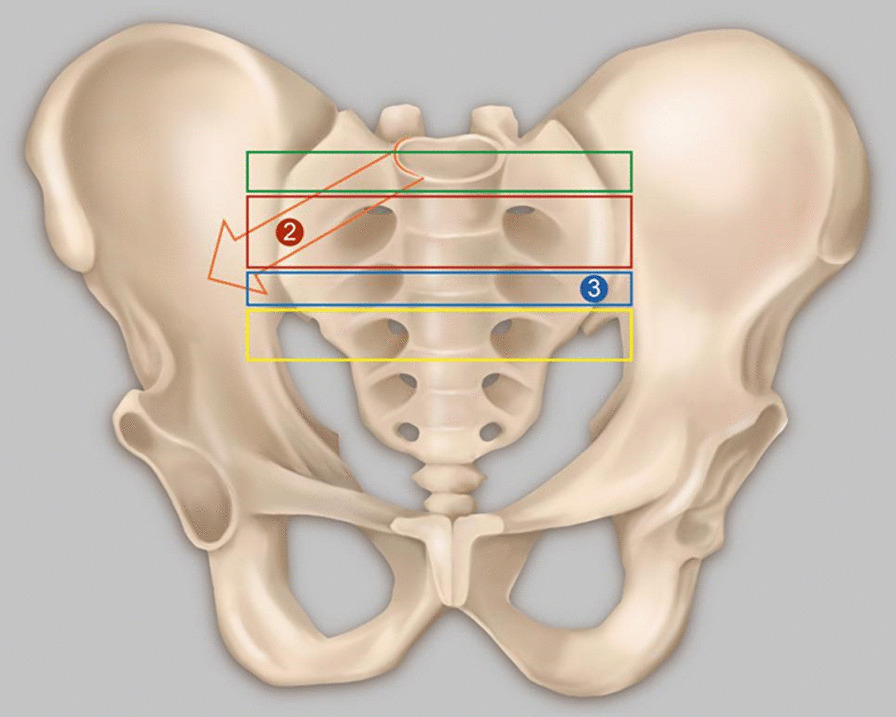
Definition and zoning of the distal and proximal sacroiliac joints. Each level of CT plain scan from the sacroiliac joint emergence to the sacral 3 foramina through five markers under the boundary was divided into four zones: sacroiliac joint emergence, the widest spacing between the sacral slope and the sacral nerve trunk canal, the disappearance of the lower boundary of the sacral 1 foramen, the disappearance of the lower boundary of the sacral 2 foramen, and the disappearance of the lower boundary of the sacral 3 foramen. The level between the beginning level of sacroiliac joint emergence and the maximum spacing between the sacral slope and the sacral nerve root canal was zone 1, etc., divided into four zones
